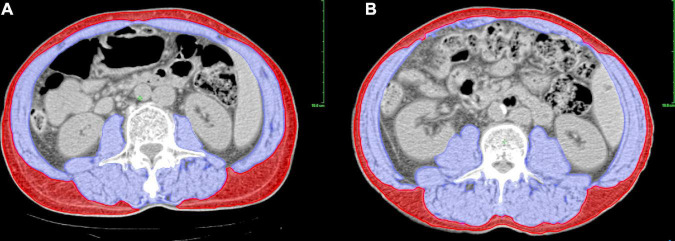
Assessment of body composition parameters using a CT-based method at L3 level. The picture shows different sarcopenic statuses in patients with the same BMI, in which blue and red zones represent the skeletal muscle and subcutaneous adipose tissue, respectively. (A) A male patient with sarcopenia (SMI = 46.52 cm^2^/m^2^, BMI = 22.50 kg/m^2^). (B) A male patient without sarcopenia (SMI = 56.19 cm^2^/m^2^, BMI = 22.50 kg/m^2^). BMI, body mass index; CT, computed tomography; L3, third lumbar vertebra; SMI, skeletal muscle index.
